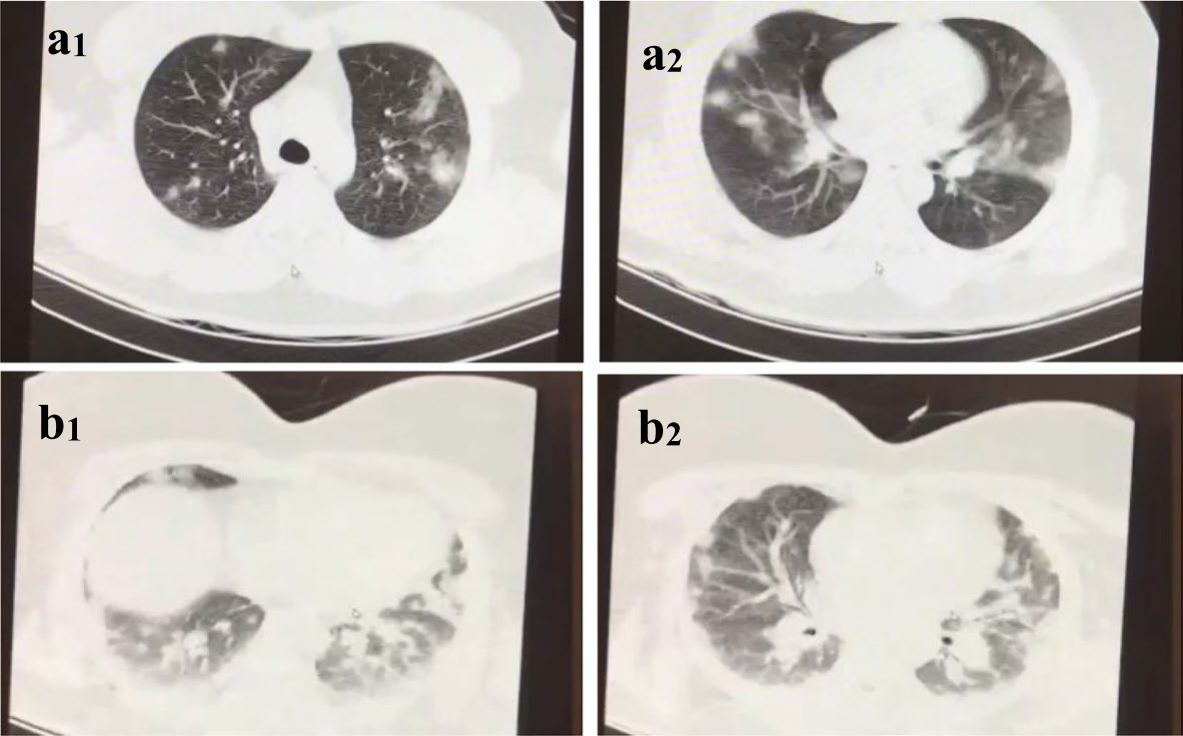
CT images of COPD patients with confirmed COVID-19. Non-severe (a_1-2_) and severe (b_1-2_)
